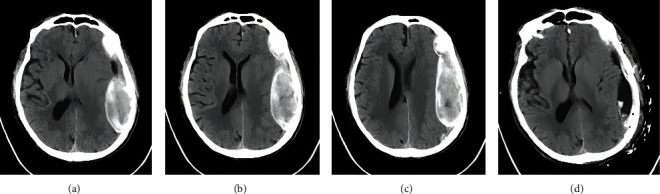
(a)–(c) show the soft tissue windows of the emergency axial head CT without IV contrast of the patient on admission, showing a huge fusiform high-density shadow in the left frontotemporal parietal region, which is very similar to the common CT findings of acute epidural hematoma. (d) shows the soft tissue window of the axial head CT without IV contrast on the first postoperative day, showing that the organized subdural hematoma was almost cleared and the compression of the brain tissue was relieved.
